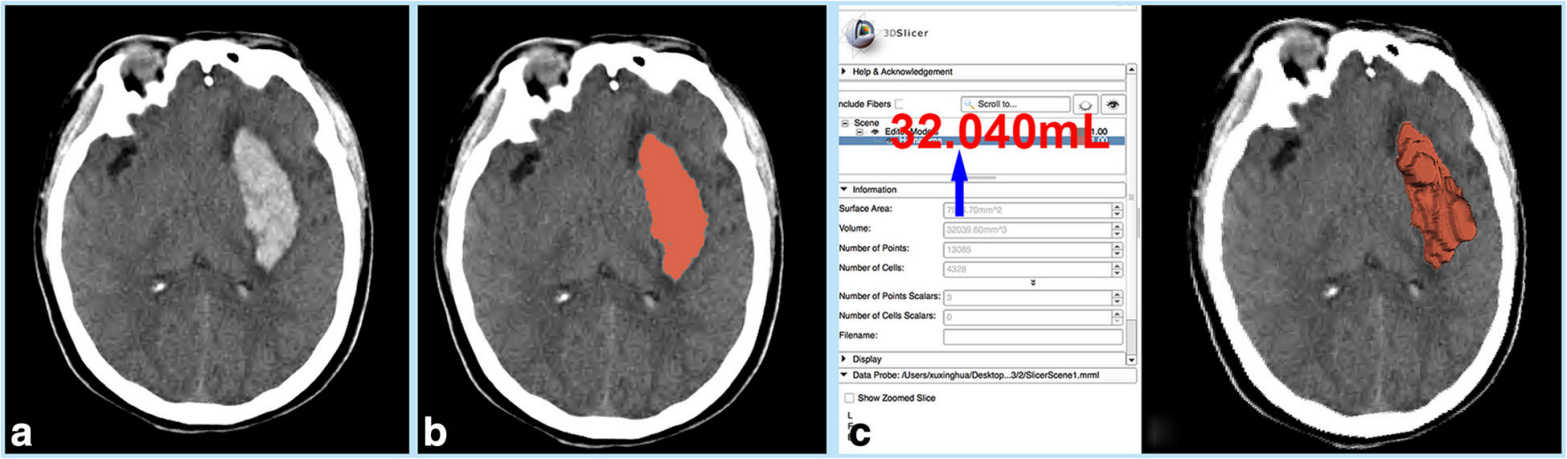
Haematoma volume measurement using the software 3D Slicer. a Preoperative brain computed tomography (CT) scan confirming left external capsular haemorrhage. b Automatic depiction of the haematoma using the threshold effect. c 3D reconstruction and volume measurement of the haematoma
